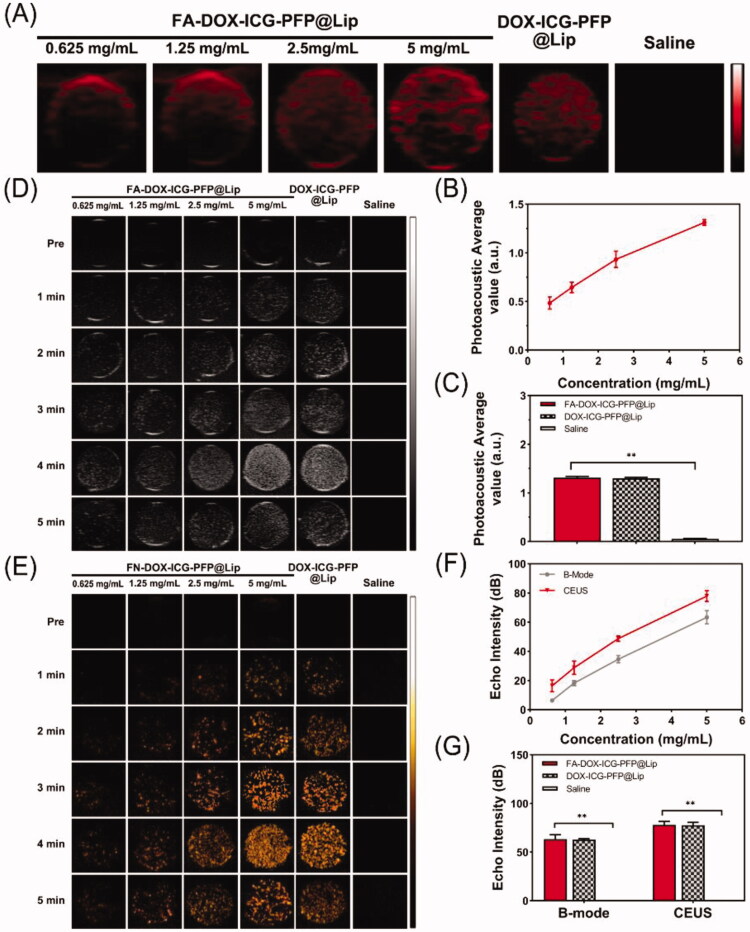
In vitro* dual-modal imaging. (A) Photoacoustic images of FA-DOX-ICG-PFP@Lip (0.625, 1.25, 2.5, and 5 mg/mL), DOX-ICG-PFP@Lip (5 mg/mL), and saline. (B) Photoacoustic values of FA-DOX-ICG-PFP@Lip (0.625, 1.25, 2.5, and 5 mg/mL). (C) Photoacoustic values of FA-DOX-ICG-PFP@Lip (5 mg/mL), DOX-ICG-PFP@Lip (5 mg/mL), and saline. (D) B-mode and (E) contrast-enhanced ultrasound images of FA-DOX-ICG-PFP@Lip (0.625, 1.25, 2.5, and 5 mg/mL), DOX-ICG-PFP@Lip (5 mg/mL), and saline with laser irradiation at 1 W/cm^2^ for 5 min. (F) Echo intensity of FA-DOX-ICG-PFP@Lip (0.625, 1.25, 2.5, and 5 mg/mL) in B-mode and contrast-enhanced ultrasound. (G) Echo intensities of FA-DOX-ICG-PFP@Lip (5 mg/mL), DOX-ICG-PFP@Lip (5 mg/mL), and saline (*p*<.01).
